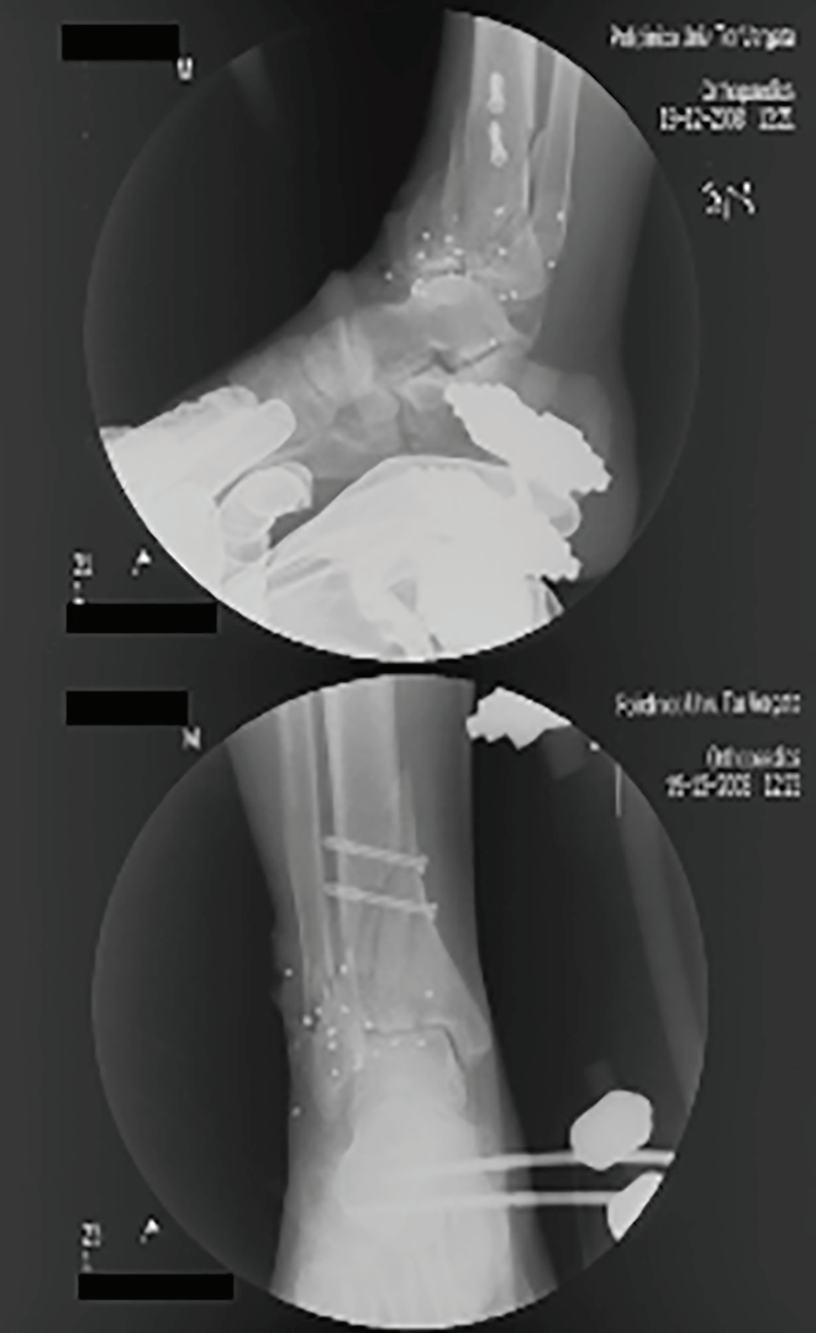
Radiographic examination: multi-fragmented fracture of distal end of tibia and also a fracture of fibula, well as numerous radiopaque foreign bodies
